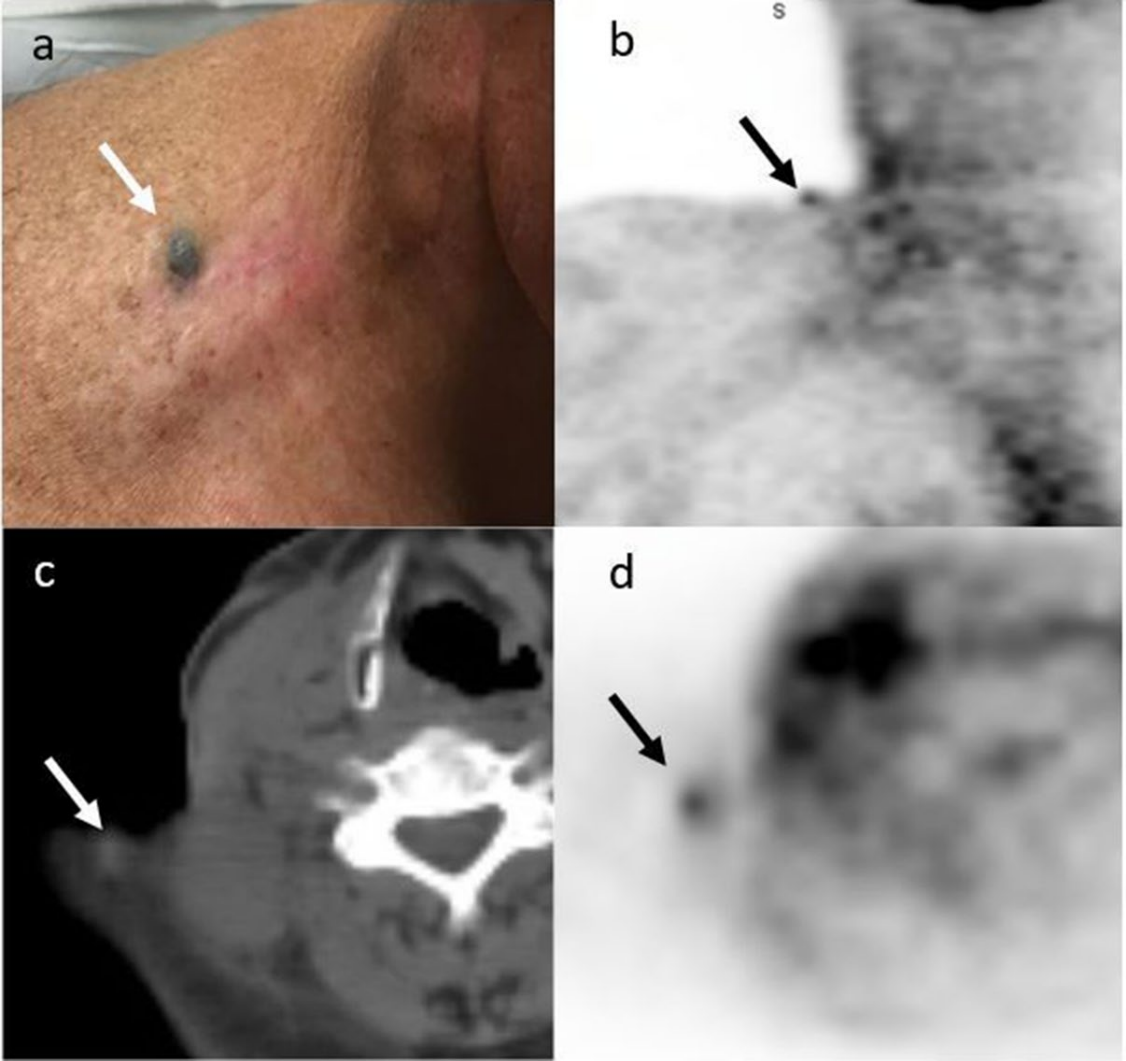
(a) Printex ink injected into subcutaneous tissue of the postoperative bed (white arrow). (b) Coronal PET image through ink injection demonstrating FDG avid lesion (black arrow). (c) On the axial CT component of the PET-CT, a focally dense area is evident corresponding to the region of the injected ink (white arrow). (d) Registered PET image to CT from (c). The area noted on the CT is FDG avid (black arrow).
